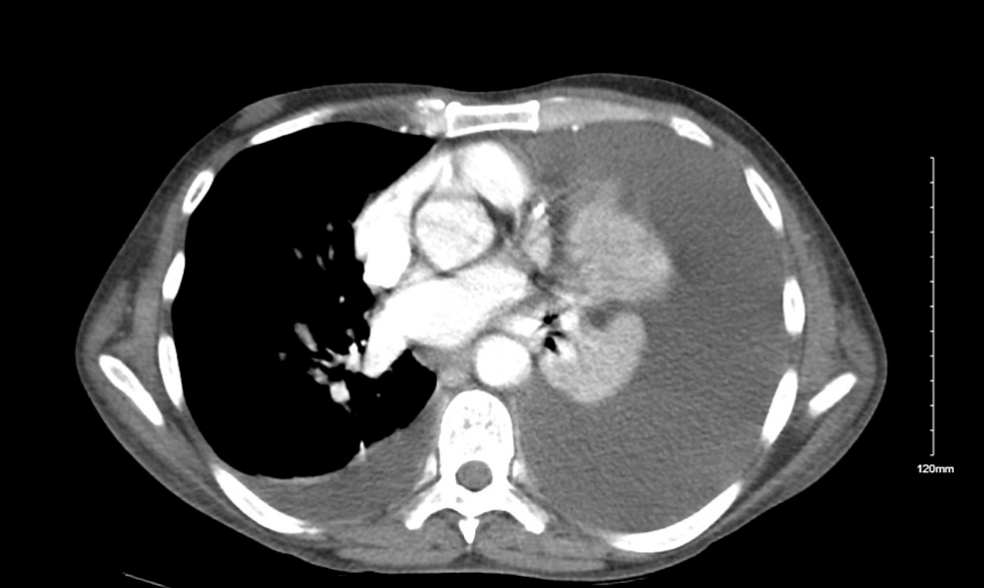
Axial section of chest CT demonstrating a massive left-sided pleural effusion and a moderate right-sided pleural effusion with compression atelectasis.
